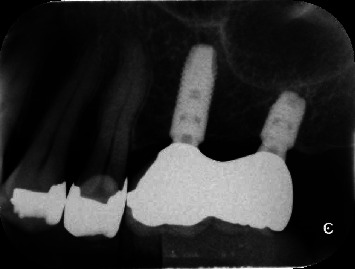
Intraoral radiographs showing after 3 years of loading. One short implant and one standard-length implant were placed next to each other.
